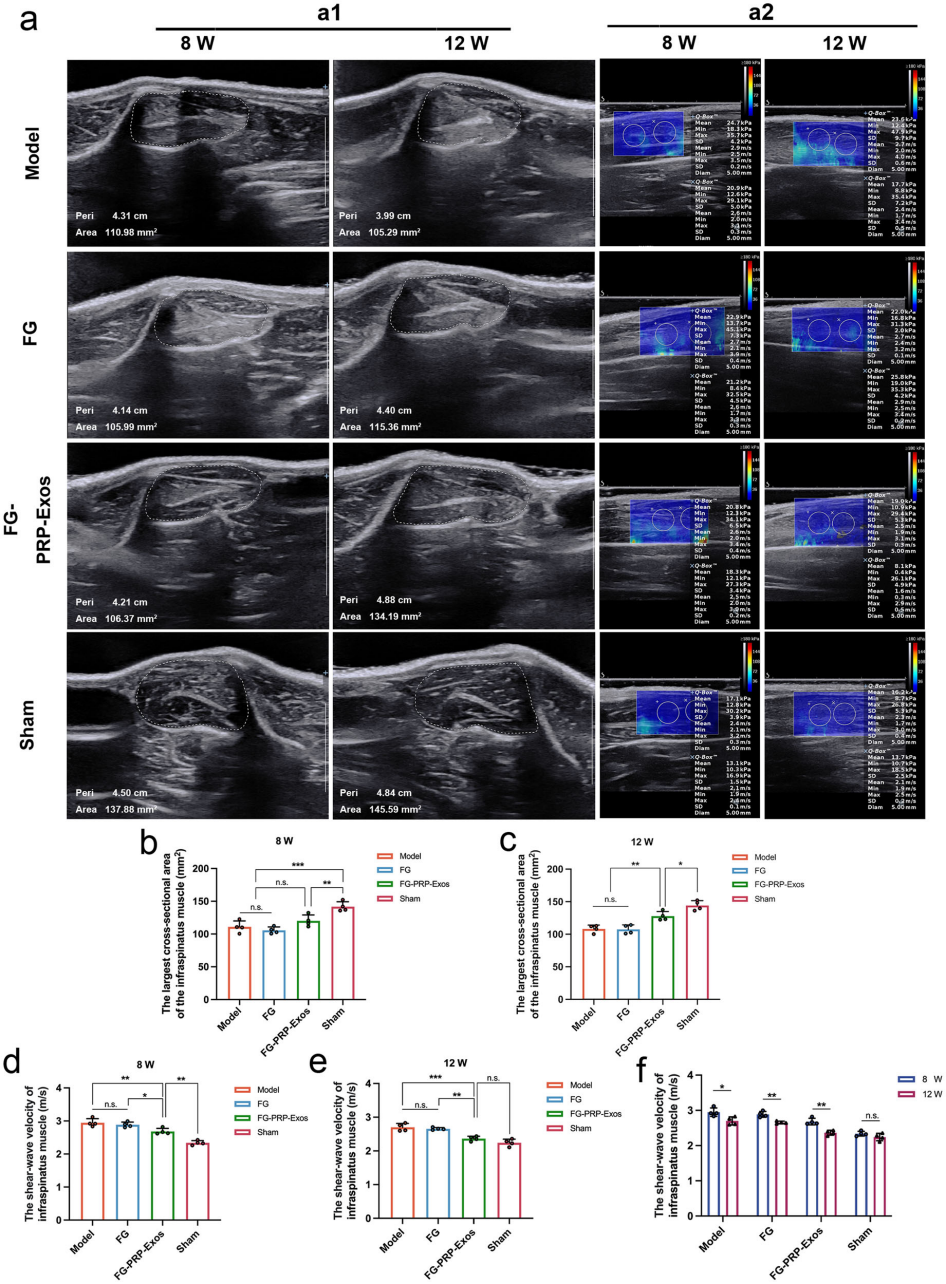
Ultrasound images of the 8 W and 12 W surgical lateral target muscles of the infraspinatus muscle. a1 Conventional ultrasound measures the muscle dimension of the largest cross-sectional area of the infraspinatus muscle, with the white dashed line showing the region of interest (ROI). a2 Shear wave elastography (SWE) image of infraspinatus muscle, ROI diameter = 5 mm. Statistical analysis was performed by calculating the maximum diameter cross-sectional area (b, c) and shear wave velocity value (d, e) of the target muscles (Each group, *n* = 4). f Comparison of 8 W to 12 W dynamic target muscle recovery in each treatment group (Each group, *n* = 4). n.s. *P* > 0.05, P* < 0.05, *P* < 0.01, P* < 0.001. All the error bars above denote standard deviation. Statistical methods, One-way analysis of variance with Tukey’s multiple-comparison test in (b–e) and two-tailed unpaired Student’s *t* test in (f).
